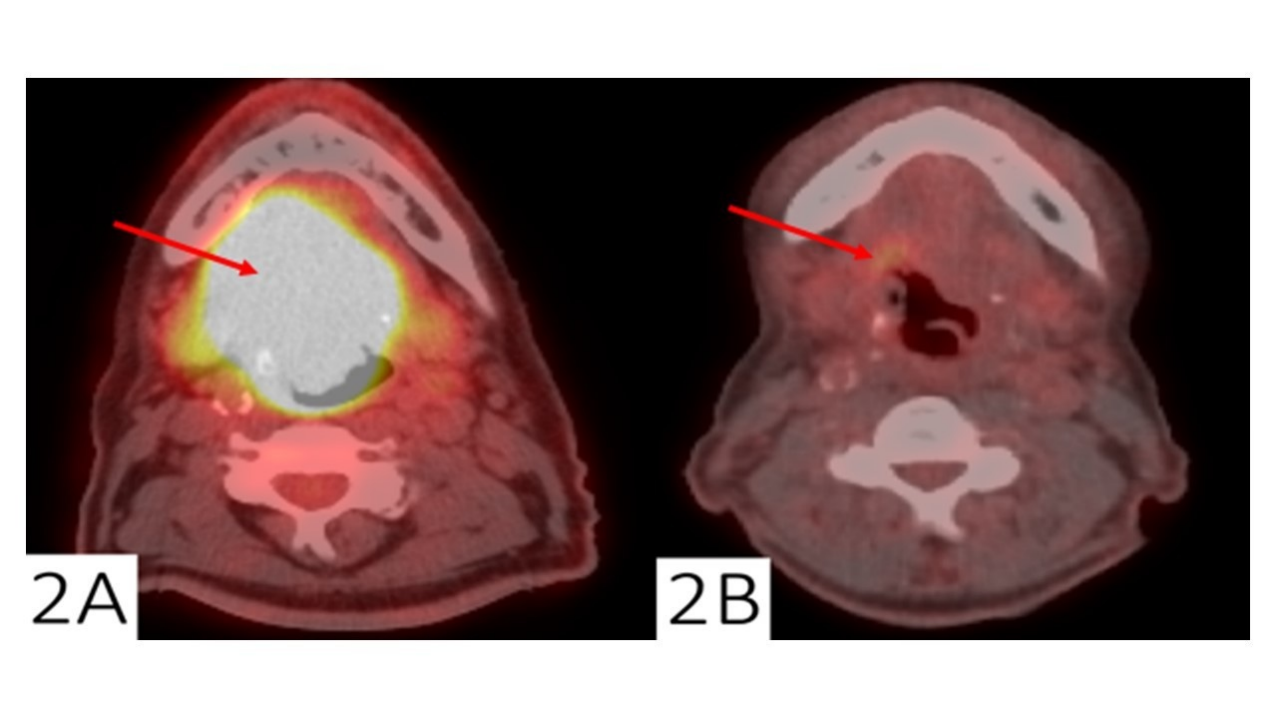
False positive post-treatment positron emission tomography/computed tomography (PET/CT) scan Pre-treatment and post-treatment positron emission tomography/computed tomography (PET/CT) for an oropharyngeal cancer. Representative matched axial PET/CT scan slices at approximately the level of the base of tongue from a patient treated with definitive chemoradiation for initially staged T4aN0M0, stage IVA, oropharyngeal cancer; (a) pre-treatment PET/CT scan with a CT mass measuring 5.7 x 5.0 cm at this level and SUVmax of 18.2 and; (b) post-treatment PET/CT scan with no obvious residual CT mass and SUVmax of 4.3
